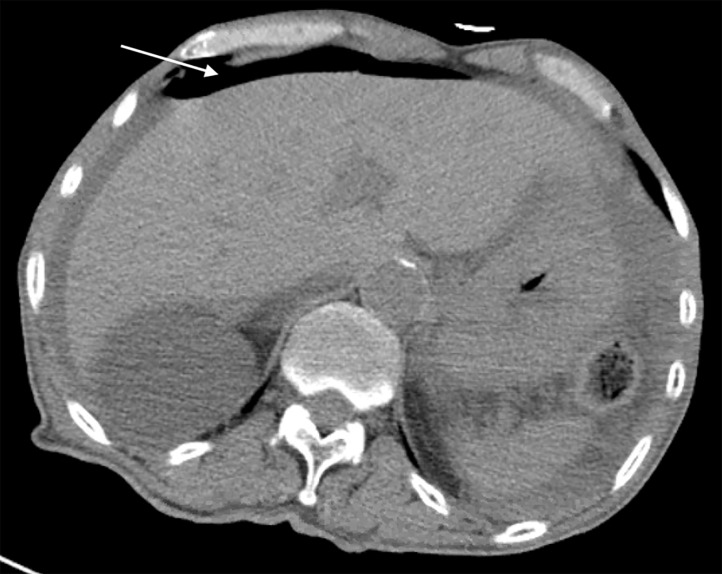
Computed tomography image from Case 2 depicting free intraperitoneal air (arrow).
